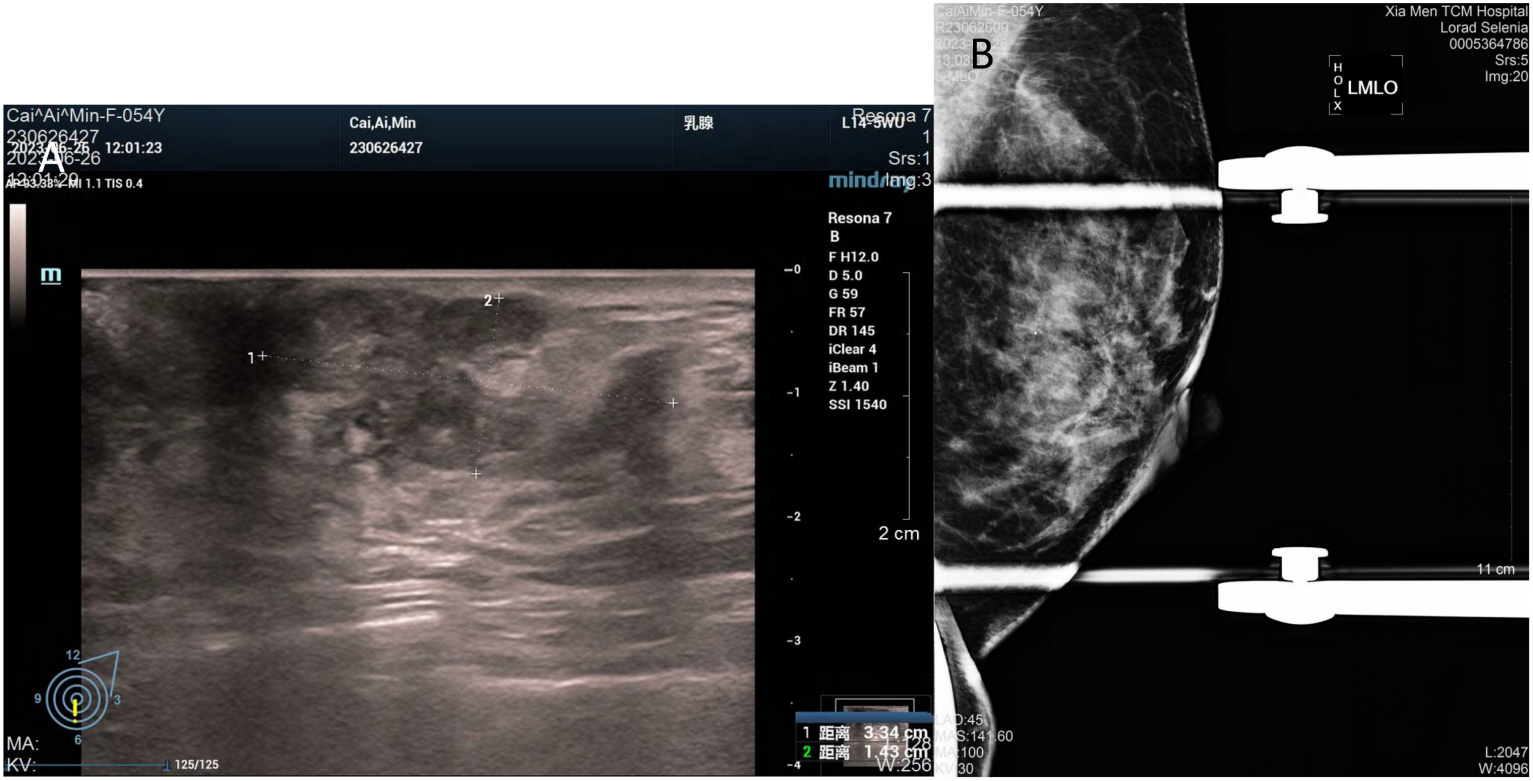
Imaging of the tumour. (A) Ultrasound shows an irregular, solid hypoechoic mass measuring 33mm×14mm with angular margins next to the nipple at 6 o’clock in the left breast. (B) Mammogram shows benign changes in both breasts, no mass was seen.
